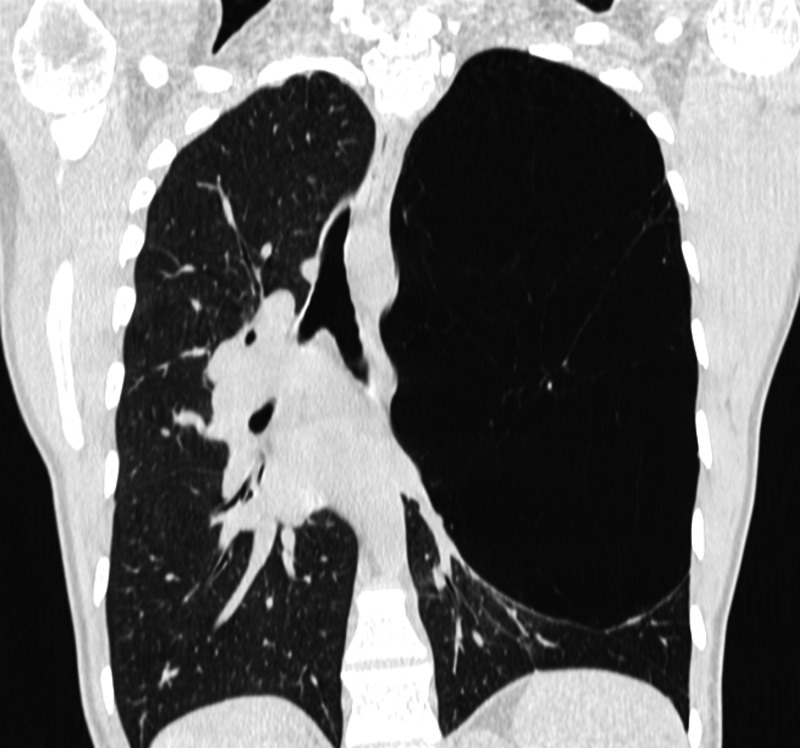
Chest computed tomography (coronal).
